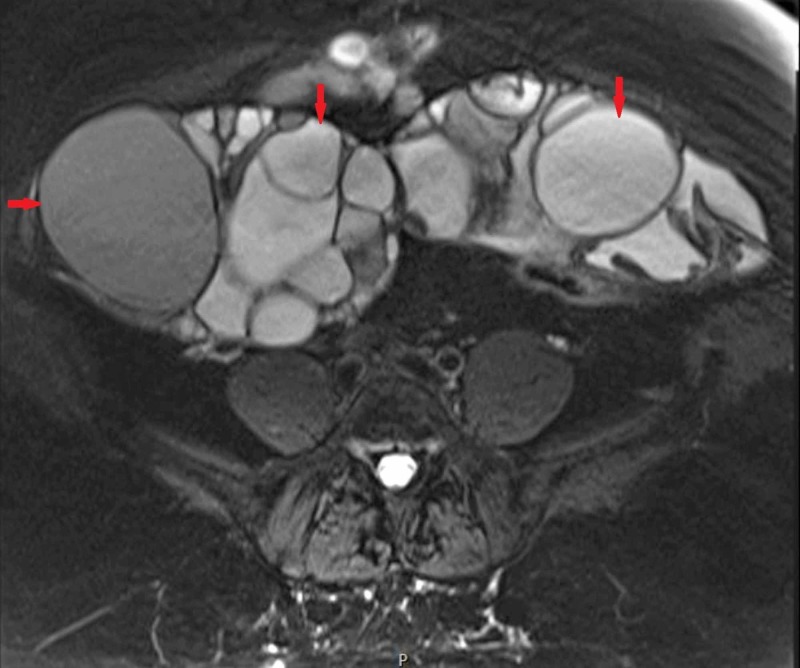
Transverse section of the patient's pelvic MRI showing severely enlarged bilateral ovarian masses with numerous cystic spaces separated by thin septations
